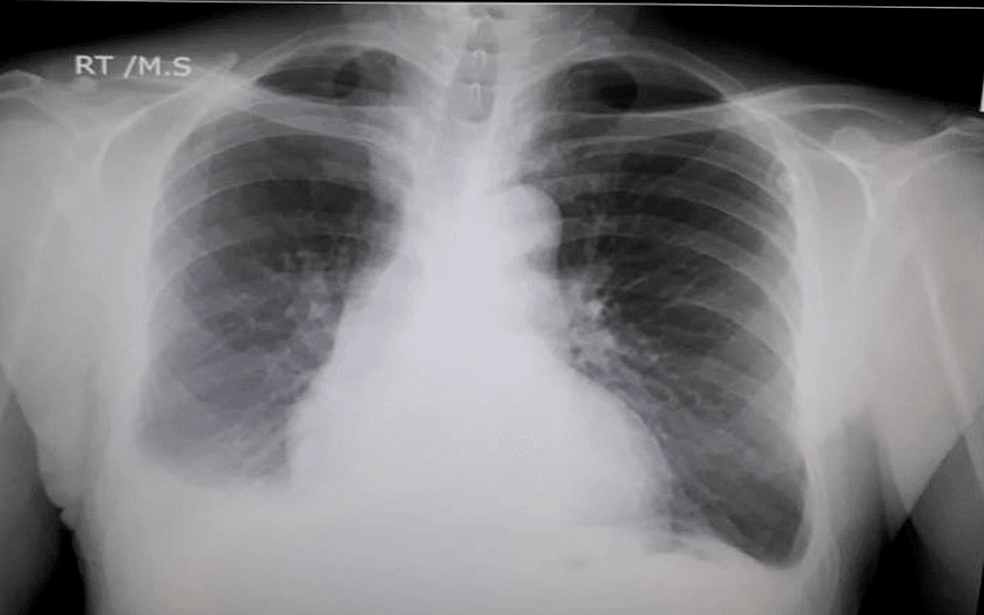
Chest radiograph PA view Shows mild right pleural effusion, mild cardiomegaly and dilated right heart cavities
